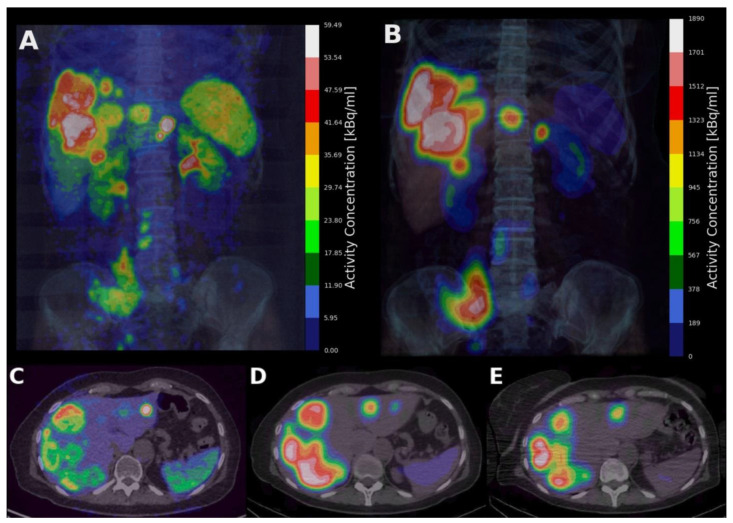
Maximum intensity projection (MIP) of a [^68^Ga]Ga-DOTA-TOC PET/CT image (A); and corresponding [^177^Lu]Lu-DOTA-TATE SPECT/CT image at the 24 h p.i. timepoint (B); axial slices of [^68^Ga]Ga-DOTA-TOC (C); and [^177^Lu]Lu-DOTA-TATE at t_24_ (D); and t_168_ (E), showing uptake in SSTR-expressing tumours. Physiologic uptake in kidneys, spleen and liver is also visible.
